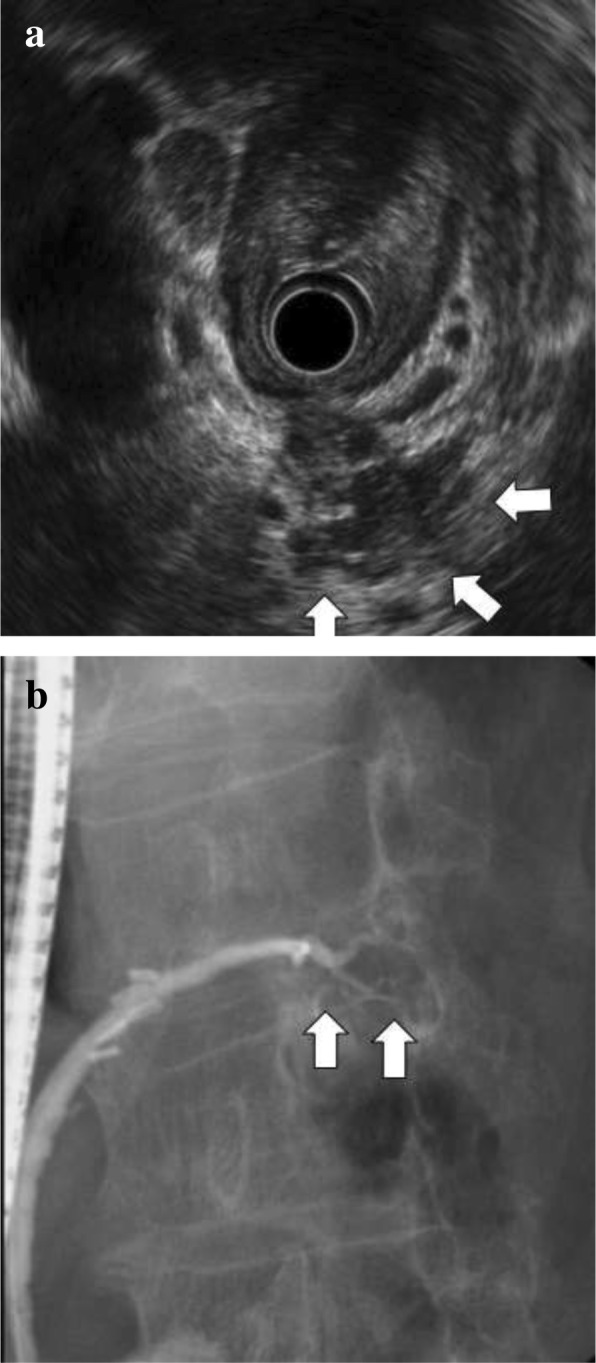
a Pre-operative endoscopic ultrasonography/retrograde pancreatography findings. Pre-operative endoscopic ultrasonography detected an adherent mass at the pancreatic tail; b endoscopic retrograde pancreatography revealed narrowing of the main pancreatic duct in the pancreatic tail
